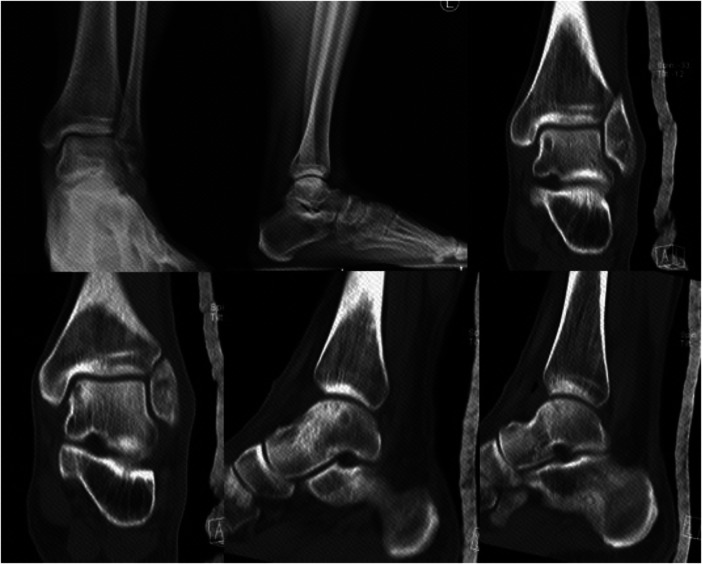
Immediately CT after surgery showed the ideal paths of retrograde drilling surgery.
